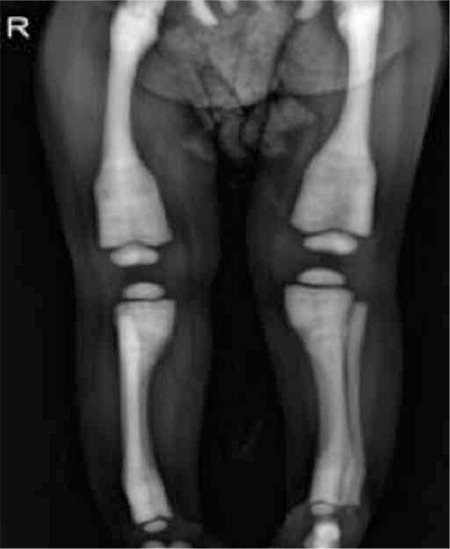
Radiograph of limbs shows Erlenmeyer flask deformity of distal femur and generalized increased bone density with the obliteration of the marrow cavity.
